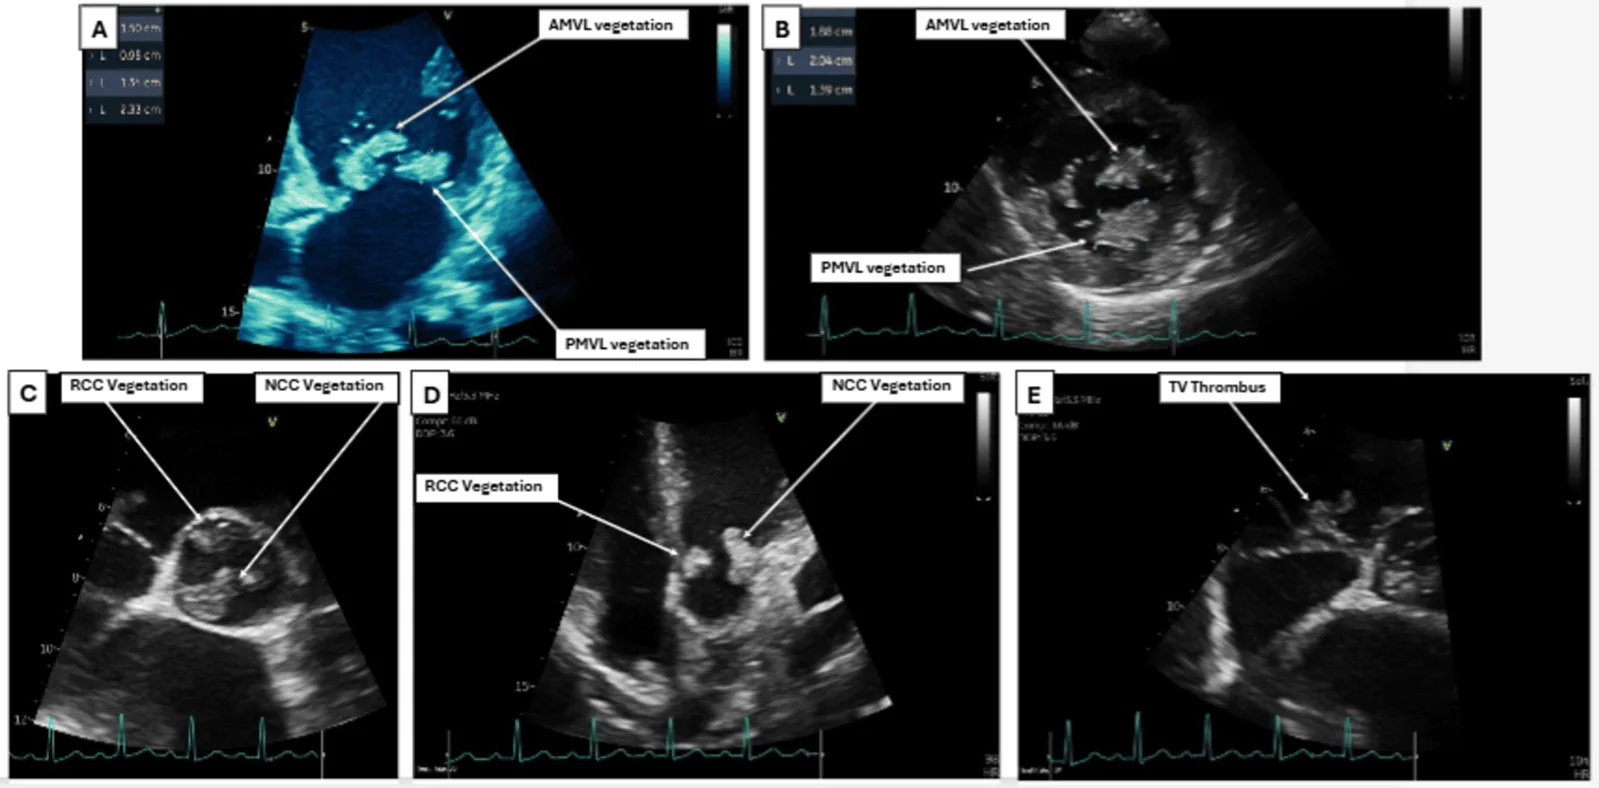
TTE images demonstrating valvular endocarditis (A) TTE A4CH view focused on the mitral valve, showing vegetations on the AMVL and PMVL. (B) TTE PSAX view at the mitral valve level, showing vegetations on the AMVL and PMVL. (C) TTE PSAX view at the aortic valve level, showing vegetations on the NCC and RCC. (D) TTE A5CH view focused on the aortic valve, showing vegetations on the NCC and RCC. (E) TTE PSAX view focused on the tricuspid valve, showing echobright material without independent mobility, consistent with thrombus. A4CH, apical four-chamber; A5CH, apical five-chamber; AMVL, anterior mitral valve leaflet; NCC, noncoronary cusp; PMVL, posterior mitral valve leaflet; PSAX, parasternal short-axis; RCC, right coronary cusp; TTE, transthoracic echocardiography; TV, tricuspid valve
